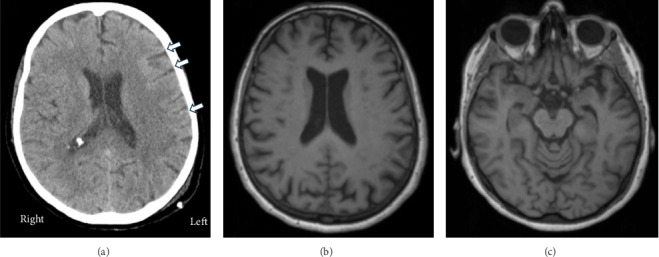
Head computed tomography taken a few hours postinjury shows very subtle subarachnoid hemorrhage on the left frontoparietal sulci (A). T1-weighted magnetic resonance brain imaging taken 2 years postinjury shows mild generalized atrophy more pronounced in the posterior frontal and parietal cortices (B) and the left hippocampus (C).
